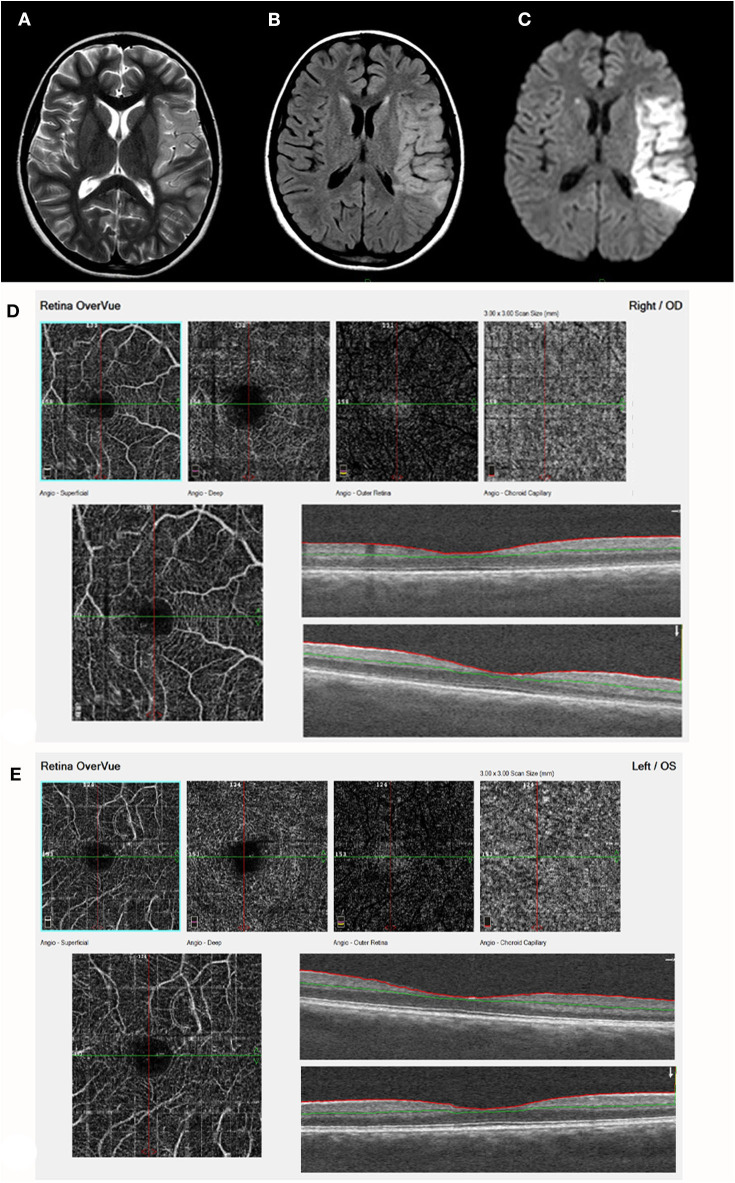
Acute stroke, left MCA territory (patient 17, Table 1). (A) T2. (B) FLAIR. (C) DWI - axial studies, acute frontal stroke with restriction on DWI. A left sylvian MCA acute infarct is visualized with restricted diffusion. The finding is visualized on a T2, FLAIR, and DWI images. (D) (right eye) and (E) (Left eye) demonstrate by ocular coherence tomography-angiography (OCT-A). Showing Segmentation of different vascular layers using OCTA: left to right: superficial plexus, deep plexus of the inner retina, outer retina (shows absence of vasculature), and choriocapillaris layer. Severe attenuation of the retinal arteries in the superficial layer is demonstrated in both eyes, Lower line: superficial retina and OCT b-scan image of the retina, showing normal retinal layers.
